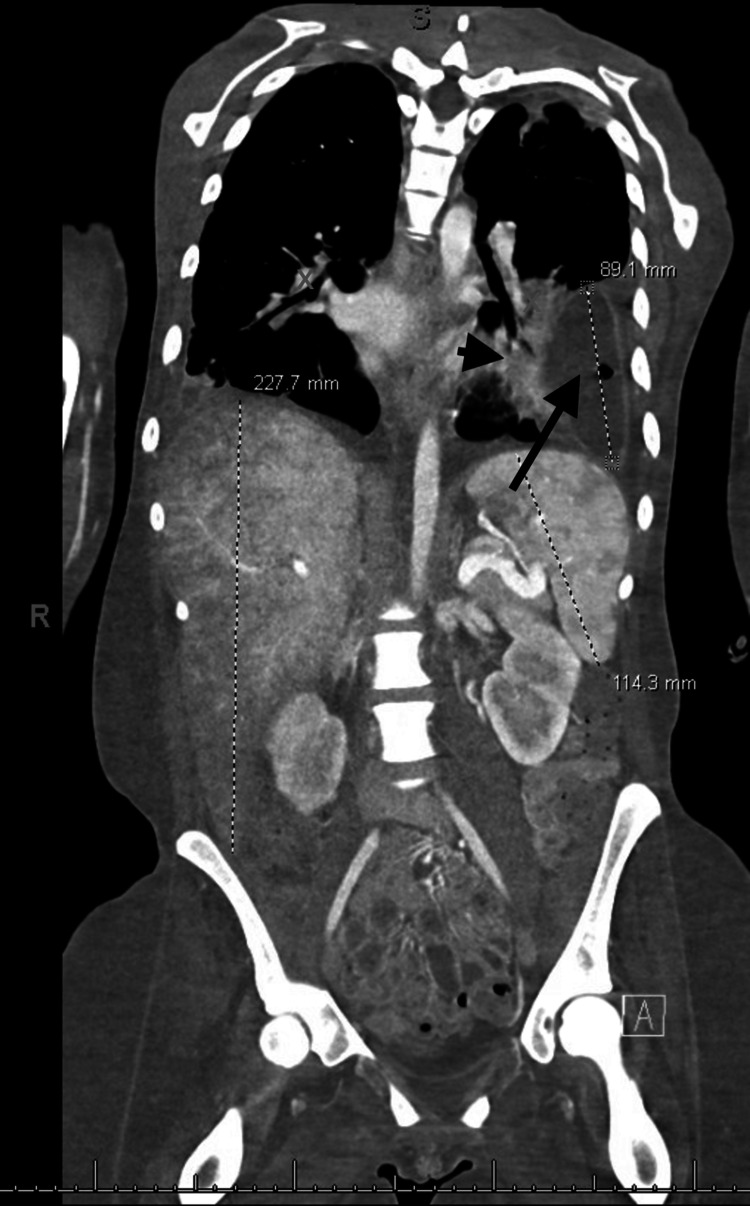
Coronal CT abdomen and pelvis with contrast showing septic emboli (arrowhead) and hydropneumothorax (arrow) consistent with empyema (measured 89.1 mm) in the left lower lung lobe.
